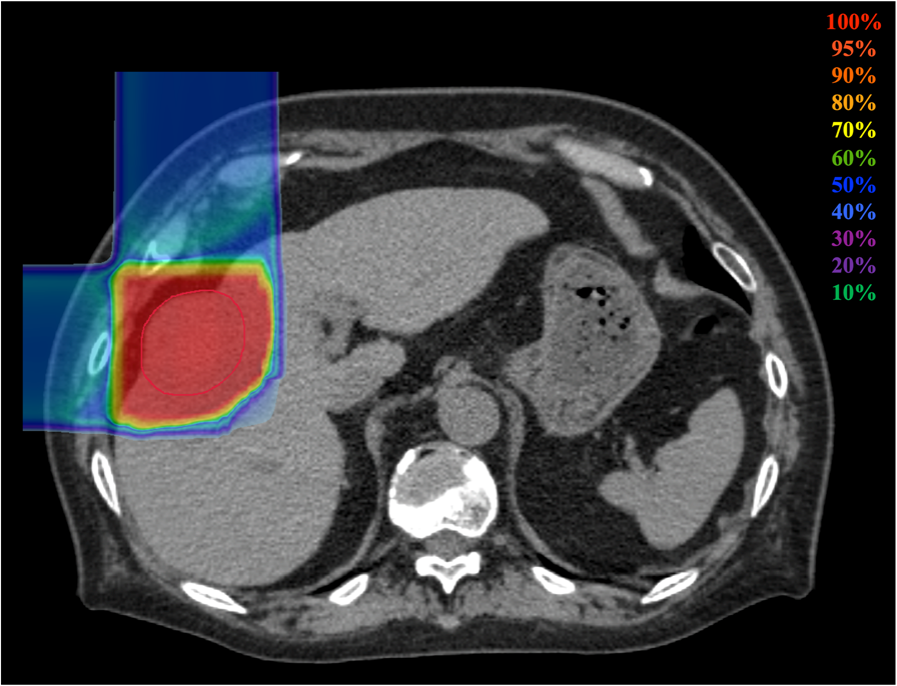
Dose distribution of C-ion RT for HCC. Isodose curves of C-ion RT are superimposed on an axial computed tomography image for the total irradiation plan. The area within the red outline is the gross target volume. Highlighted are 100% (red), 95% (light red), 90% (orange), 80% (light orange), 70% (yellow), 60% (green), 50% (blue), 40% (cyan), 30% (light purple), 20% (purple), 10% (light blue) isodose curves (100% was 60 Gy [relative biological effectiveness])
